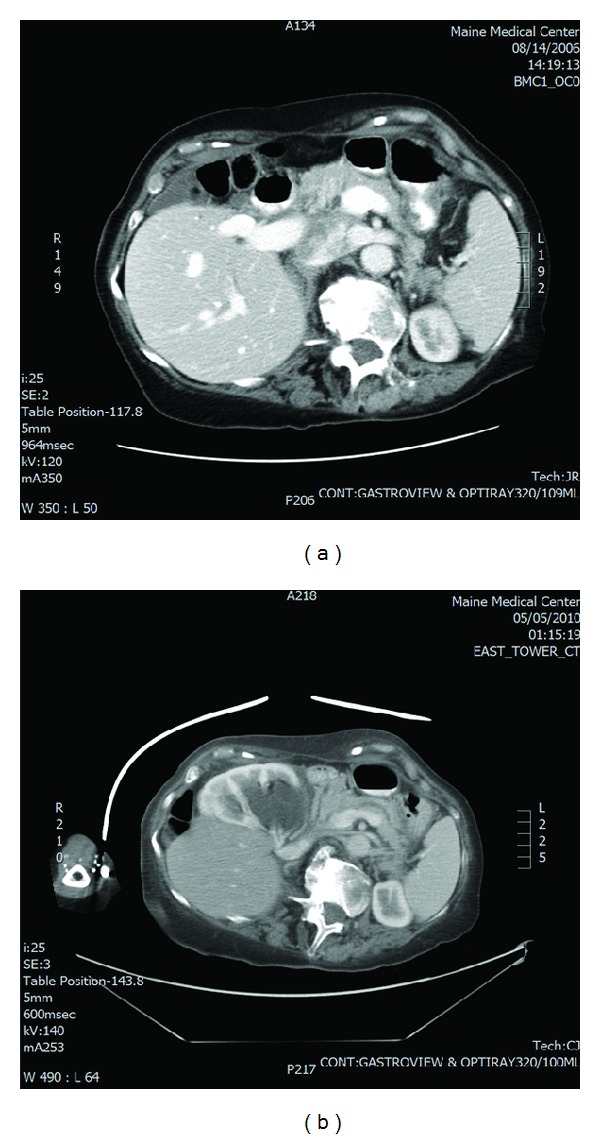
Previous CT scan from 2006 (a) and CT scan from 2010; (b) patient presentation in the emergency department depicting new anterior position of right kidney.
